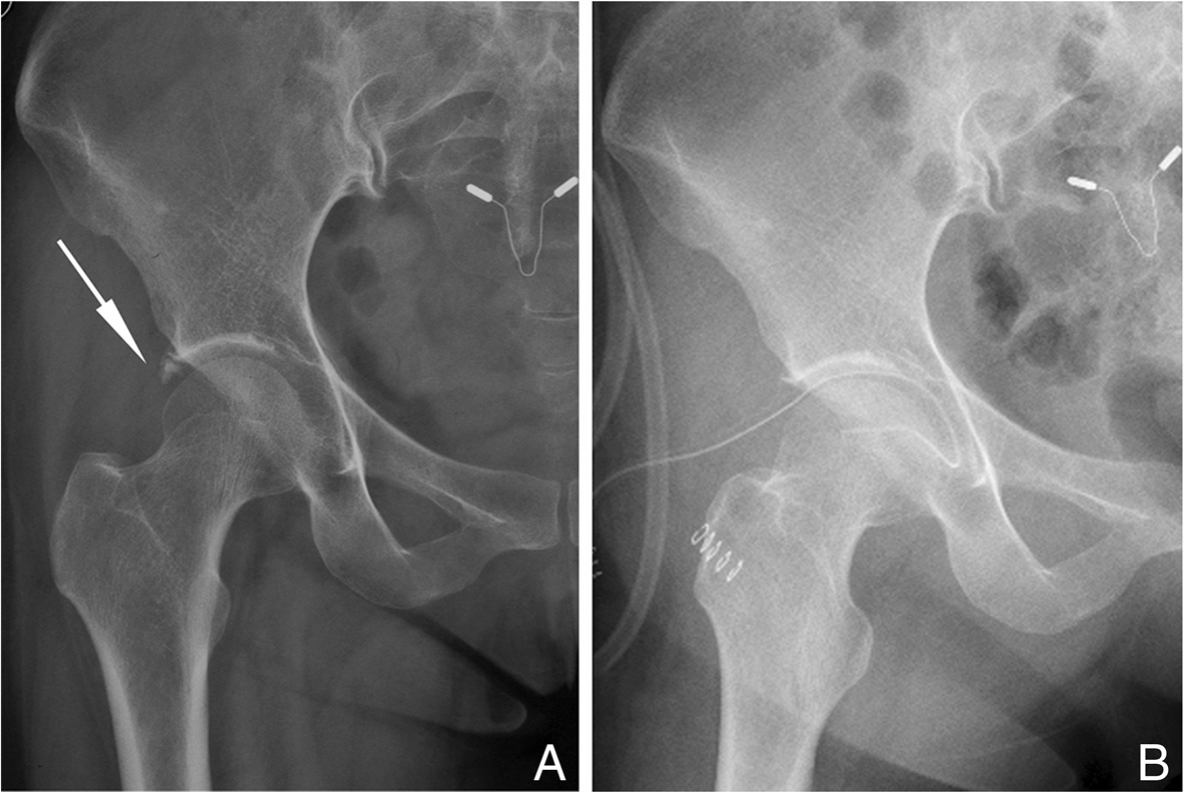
Case 2 radiographic data. (A) Preoperative radiographic anteroposterior view of the right hip with calcific densities located near the superior tip of the acetabulum (arrow) in case 2. (B) Radiographic anteroposterior view presented complete removal of calcification at postoperative day 1 in case 2.
